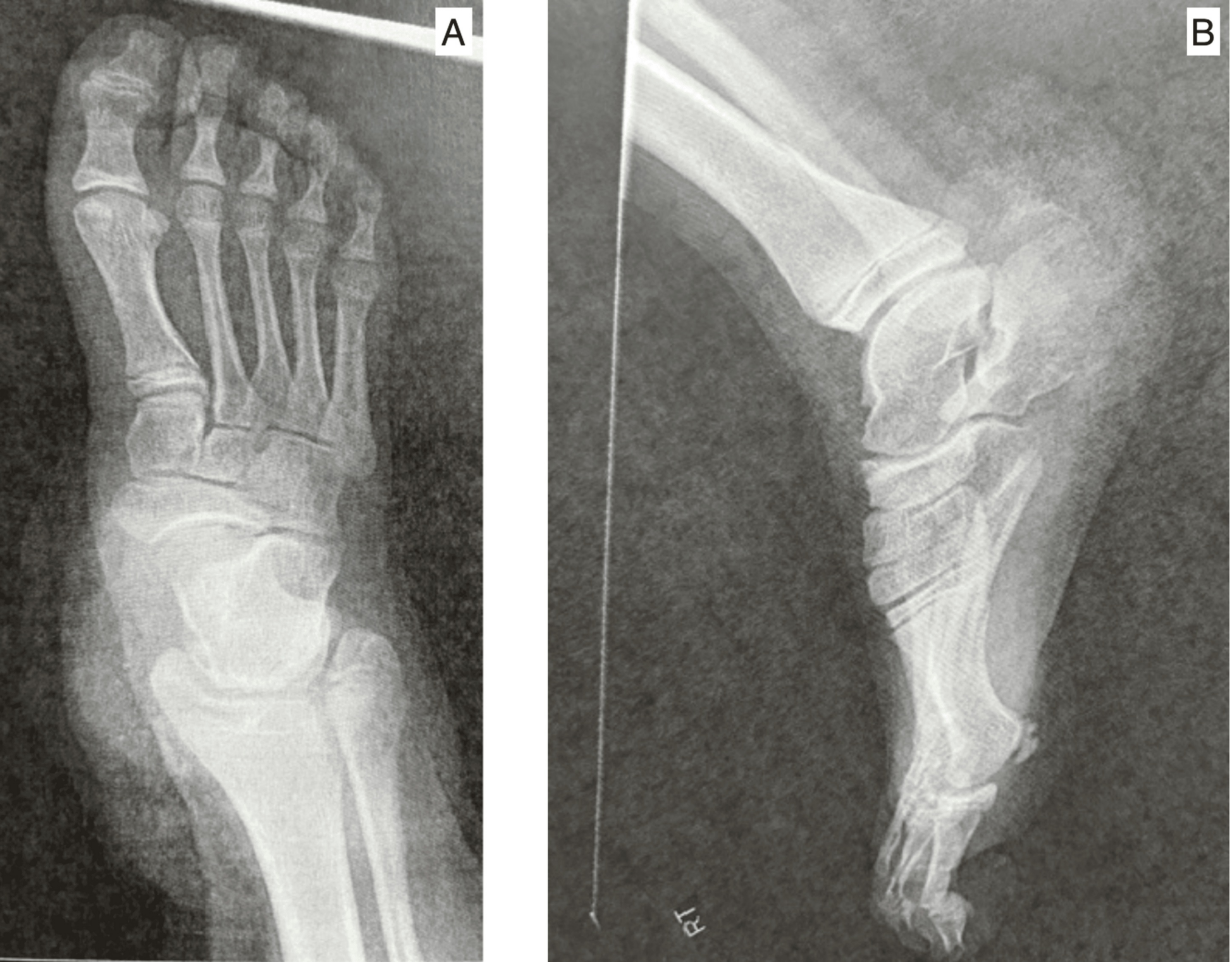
(A) Anteroposterior and (B) lateral radiographs of the right foot showing calcaneal tuberosity amputation and associated posterior soft tissue defect.
